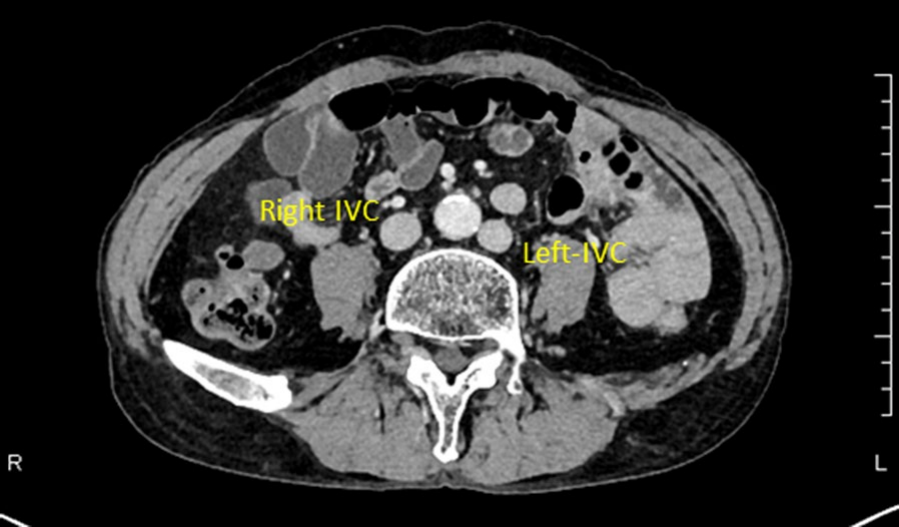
Preoperative computed tomography demonstrating a double inferior vena cava
